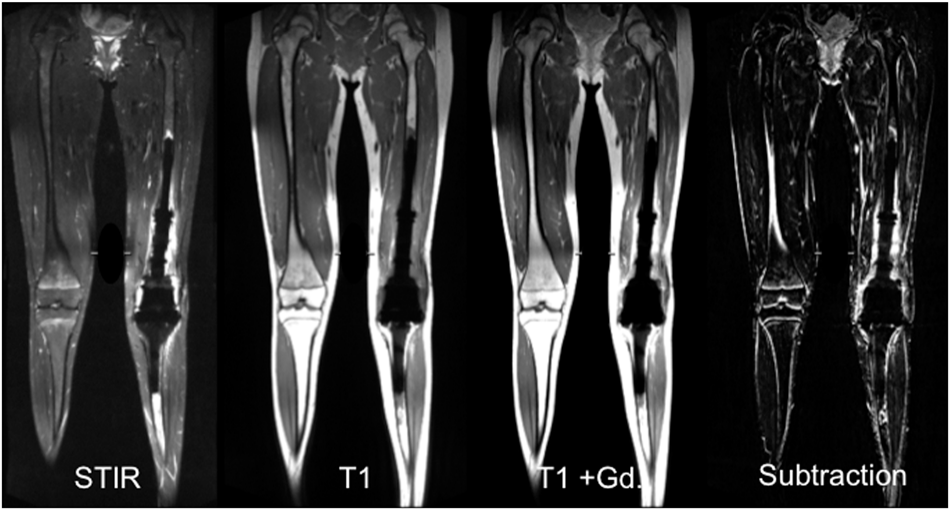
Example of the large size of the field of view. A Large field of view is needed for postoperative follow-up MR imaging in tumor patients. MR imaging was performed in a young, female patient (15 years old) after resection of an osteosarcoma at the left distal femur and implantation of a combined femoral and tibial replacement. Pulse sequences presented include a coronal STIR sequence and a coronal T1-w sequence without and with contrast application and a subtraction image, all using view-angle tilting and slice-encoding metal artifact correction with 6 slice encoding steps.
